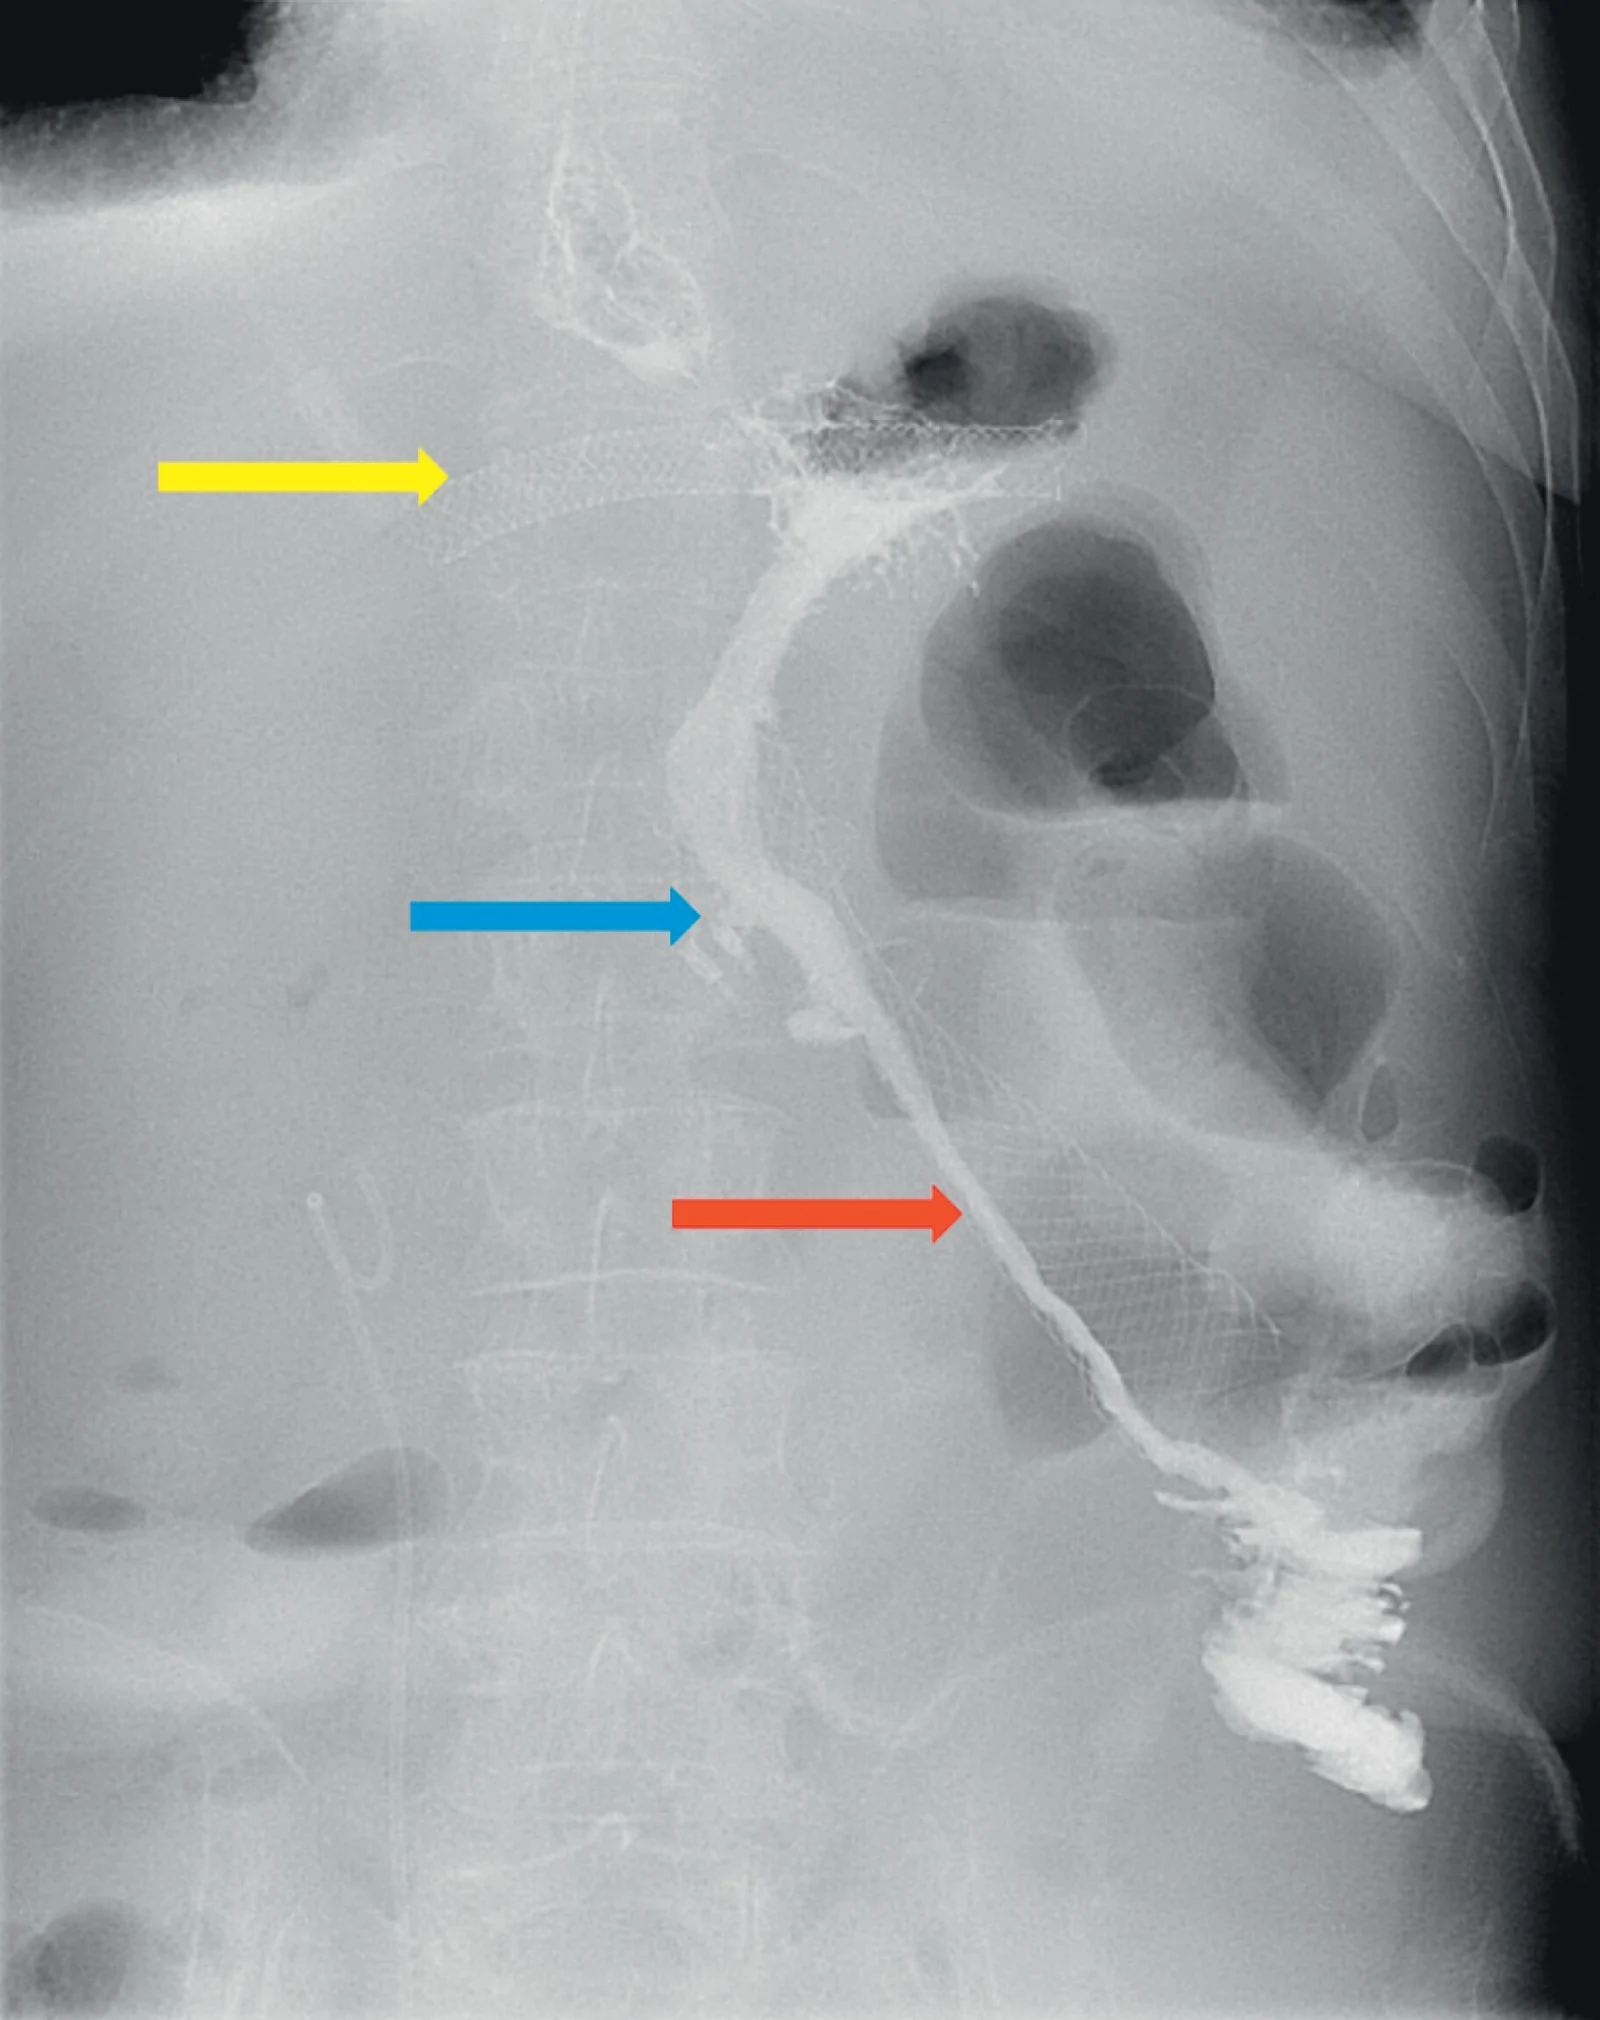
X-ray after the oral agent showing all three stents. Yellow
